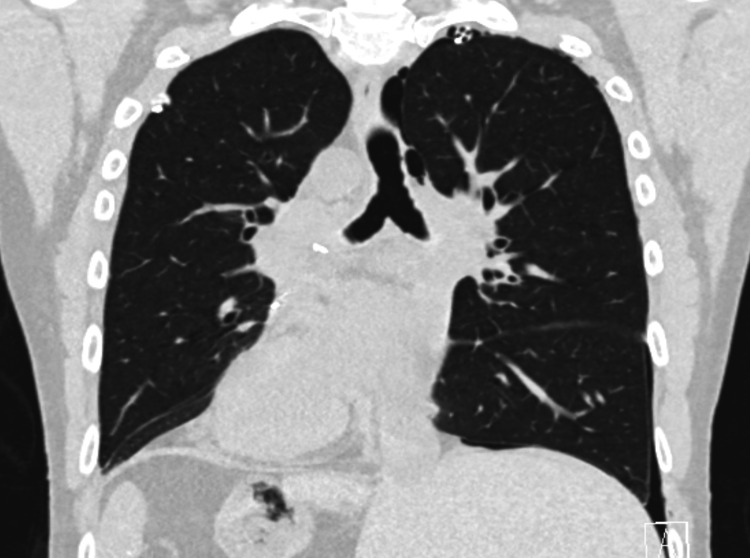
CT chest demonstrating transplanted lungs in the patient with Kartagener's syndrome
